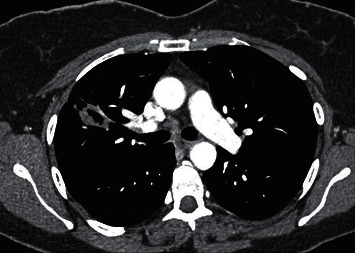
CT thorax axial view showing the cavitary lesion in the right upper lobe of the lung.
